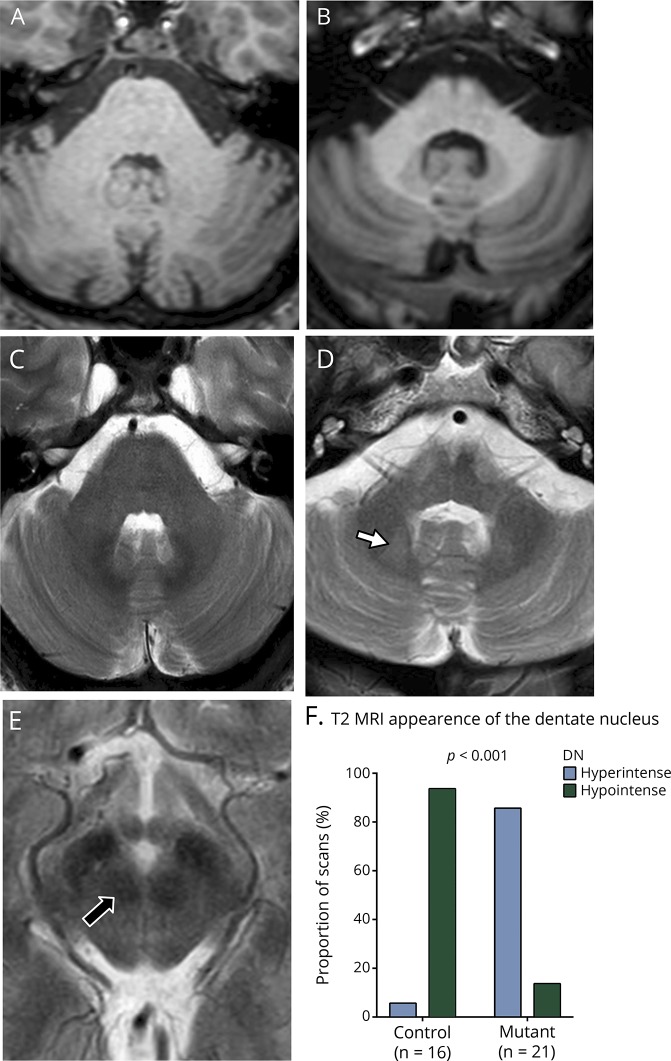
MRI of the brain in SPG7 cases shows T2 hyperintensity of the dentate nucleus (A) T1 axial image across the dentate nucleus (DN) of a control case. (B) T1 axial section through the DN in a patient with c.1529C>T homozygous mutation. (C) T2-weighted axial image of the same control and (D) T2 axial section through DN in the same patient with c.1529C>T homozygous mutation, which demonstrates hyperintense DN (solid white arrow) compared to the normal-appearing white matter. (E) T2-weighted axial image of the same patient, which demonstrates the red nucleus (RN). The RN appears hypointense compared to normal-appearing white matter in all *SPG7* and control cases (solid black arrow with white border). (F) The observation of hyperintense T2 signal of the DN was significantly more frequent in the SPG7 patients compared to the control cases (*p* < 0.001, χ^2^ test value 25.7649).
